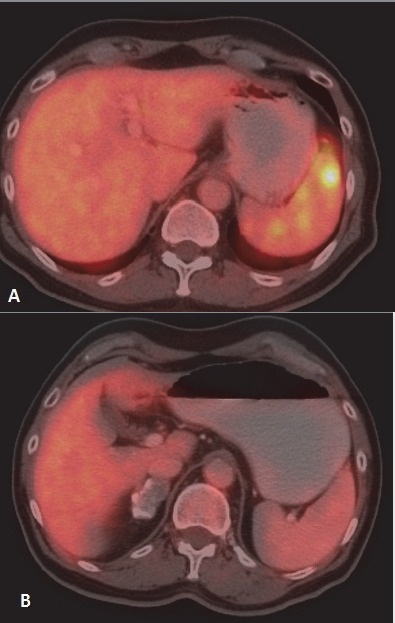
Same patient as in Fig 7. FDG avid focal lesion in spleen (A) shows complete metabolic as well as anatomical response in post treatment scans (B)
